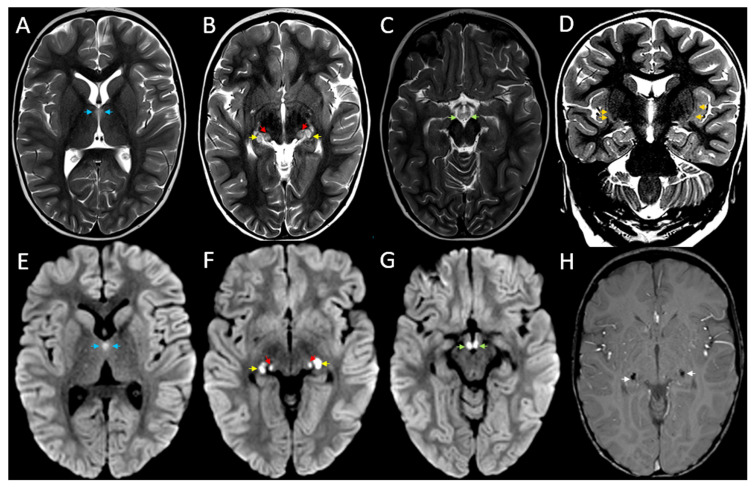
Bilateral symmetrical MRI findings in acute necrotising encephalopathy type 1. The 3T MRI images including axial T2 weighted imaging (A–C), coronal T2 weighted imaging (D), axial diffusion weighted imaging (E–G), and axial susceptibility weighted imaging (SWI) (H). There is T2 hyperintensity and corresponding diffusion restriction demonstrated within the bilateral ventromedial thalami ((A,E), blue arrows), lateral geniculate bodies ((B,F), yellow arrows), subthalamic nuclei ((B,F), red arrows), and mamillary bodies ((C,G), green arrows). T2 hyperintensity is also demonstrated within the bilateral claustrum ((D), orange arrows). The lateral geniculate bodies show evidence of haemorrhage on SWI ((H), white arrows).
